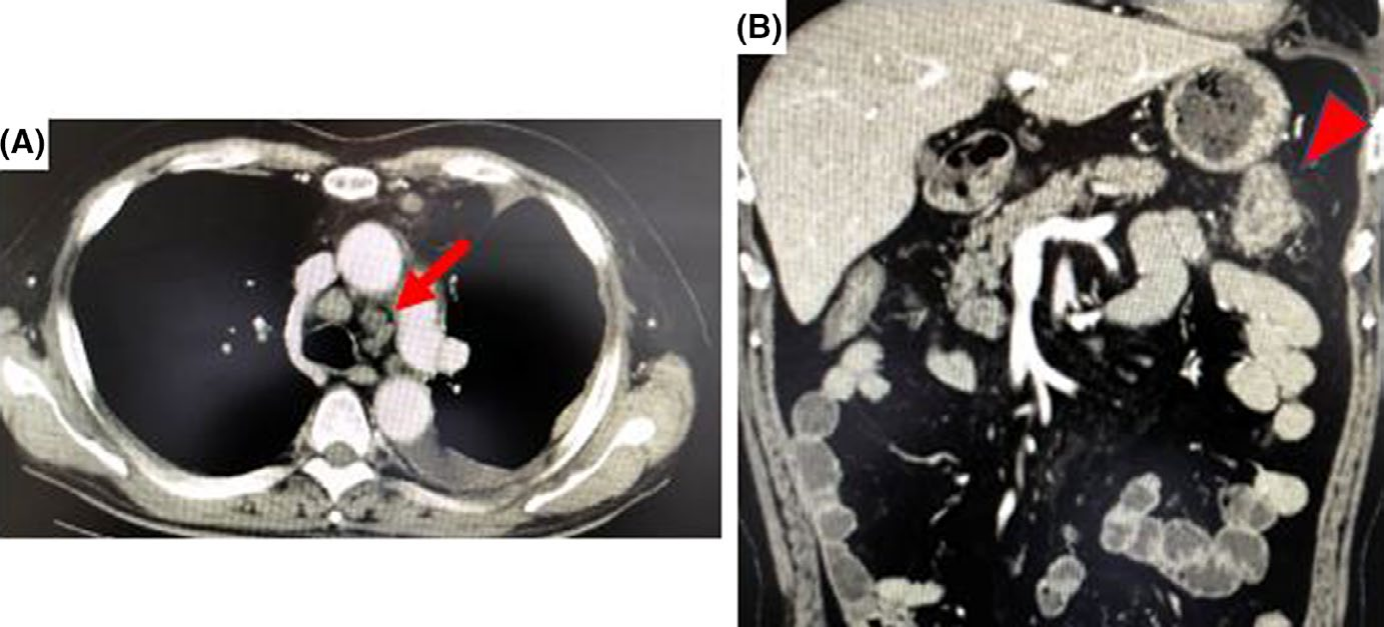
Contrast computed tomography finding. A, Swollen lymph nodes in the left hilum of lung (arrow) and left pleural effusion. B, Thickening of the wall of transverse colon (arrow head) indicating the tumor location and some nearby swollen lymph nodes
